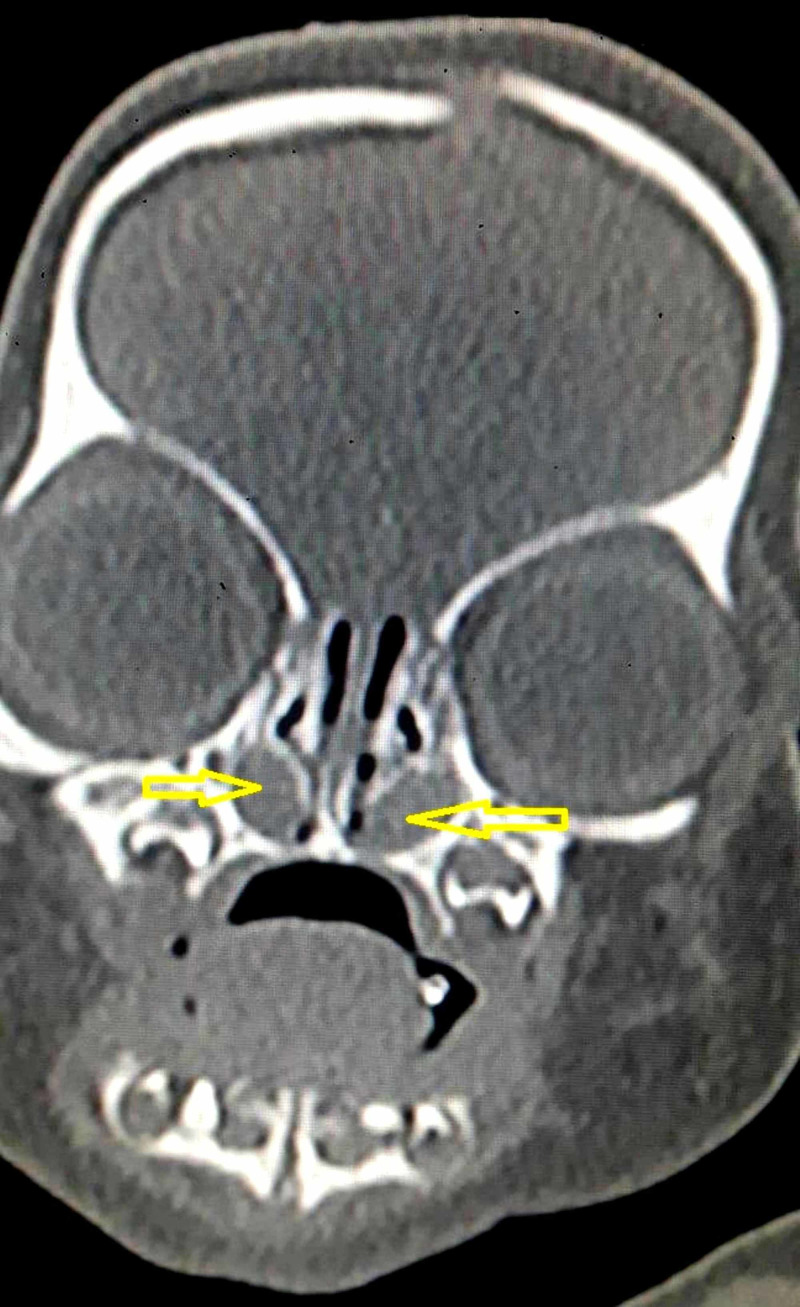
Non-contrast computed tomogram coronal section showing hypodense cystic mass in bilateral nasal cavity (yellow arrows), inferolateral to inferior turbinate.
